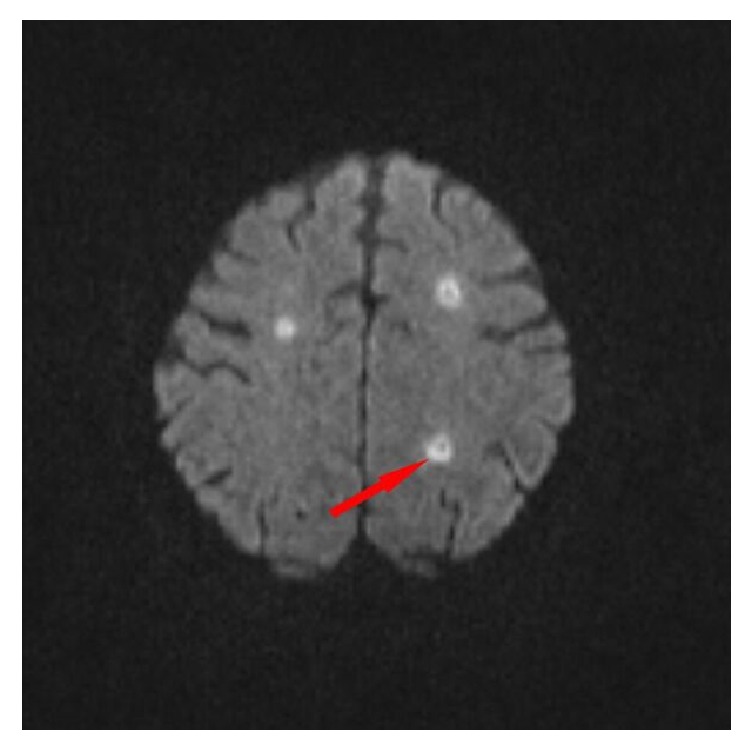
MR imaging of the cranium with multiple small rotund lesions in the sense of septic emboli (red arrow).
